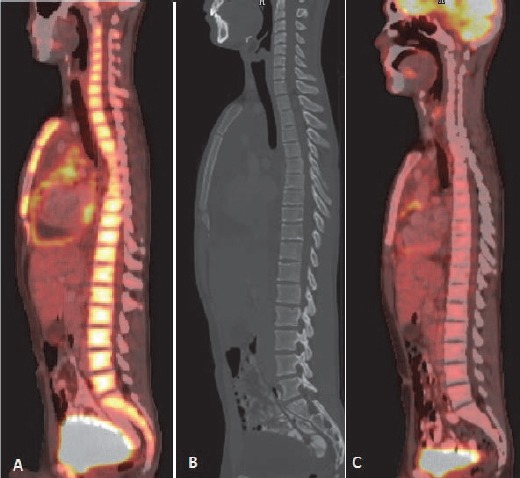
Diffusely increased radio tracer uptake seen in visualised axial skeleton post chemotherapy suggestive of marrow hyperactivation (A). CT scan shows no cortical abnormality (B). Follow up scan shows normal marrow uptake of radio tracer (C).
